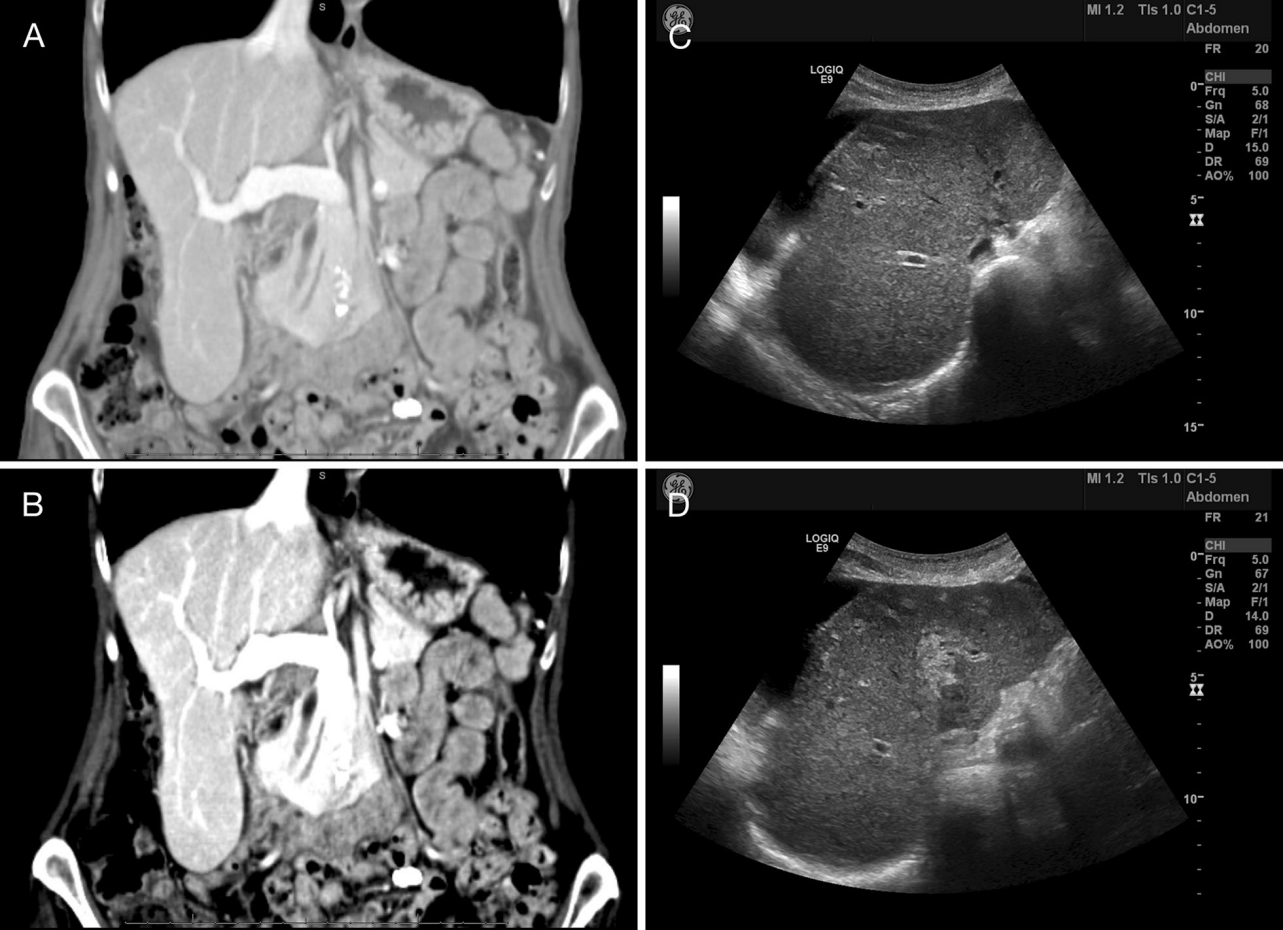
Findings on imaging. Computed tomography (CT) of the patient’s abdomen (A—abdominal view, and B—liver view) revealed a prominent Riedel lobe of the liver, with no evidence of obstruction, thrombosis, or malignancy. Mild changes of sigmoid diverticulosis were seen, with no evidence of acute diverticulitis. A repeat CT revealed mild ascites localized to the right upper quadrant, and the liver edge appeared to have a slightly nodular contour, a non-specific finding in this particular case, but which in general may suggest cirrhosis. Abdominal ultrasound (C, D) revealed a heterogeneous echotexture of the liver with regions of increased echogenicity in the right lobe, a non-specific finding which may be seen in hepatitis
